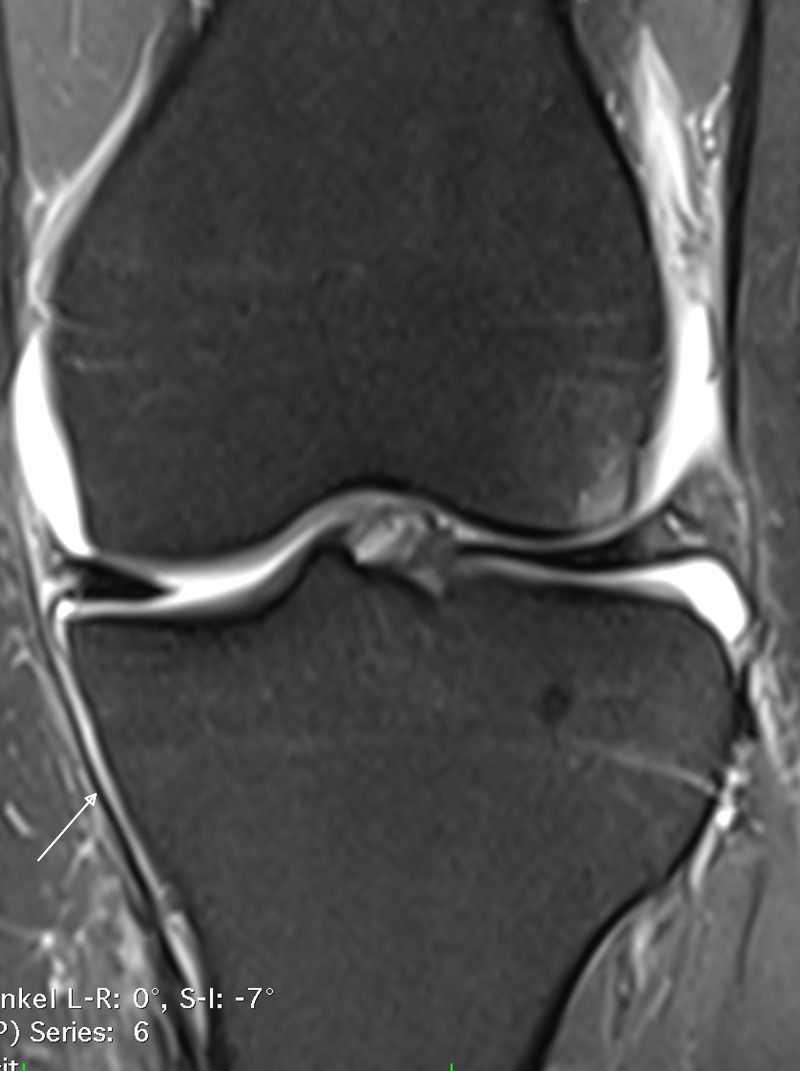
Magnetic resonance imaging of the left knee for patient 2 The T2 weighted image shows the intact superficial band of the MCL (white arrow).
